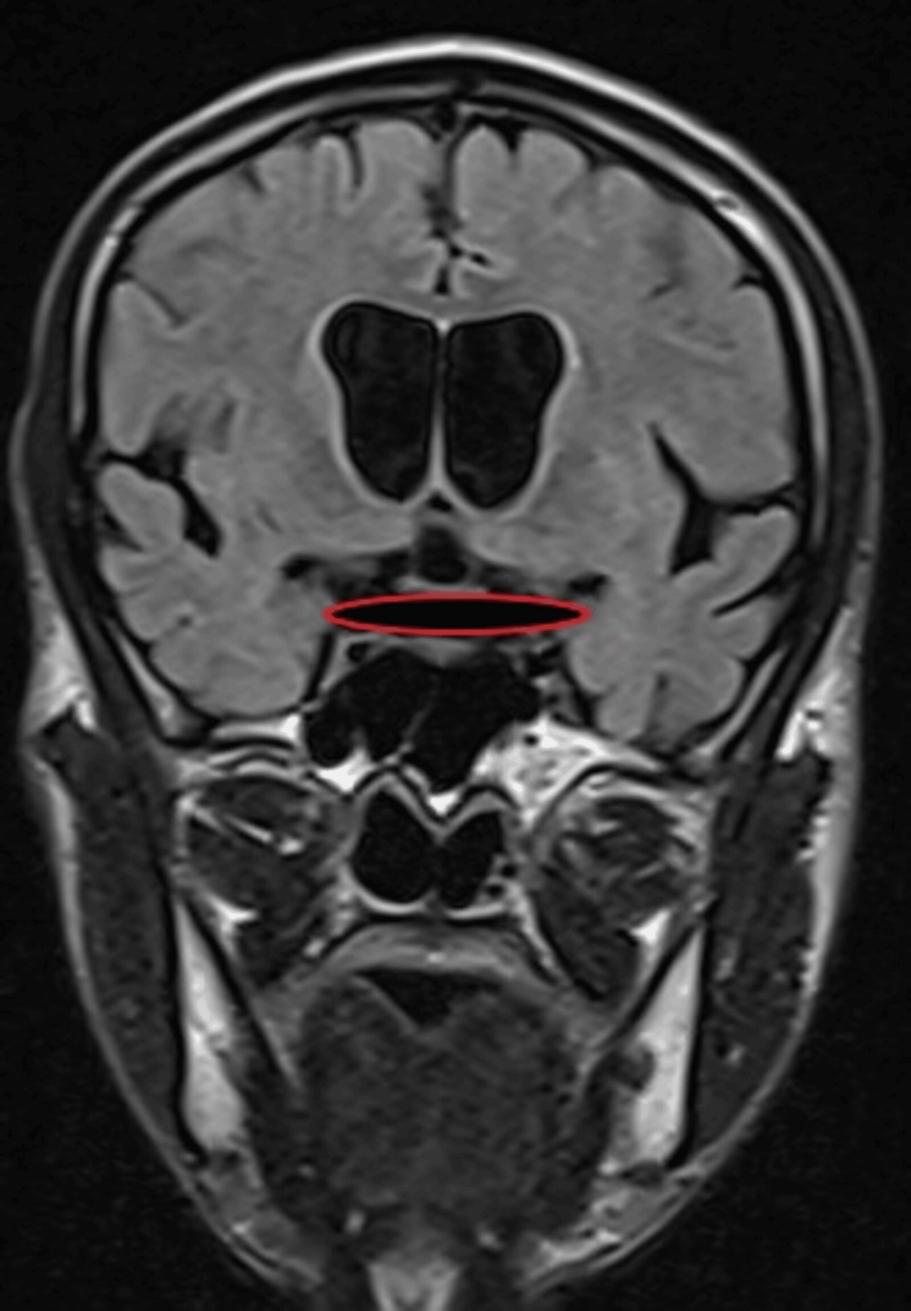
MRI of the brain in T2 FLAIR coronal section showing optic chiasma atrophy (red circle). MRI: Magnetic resonance imaging; FLAIR: Fluid attenuated inversion recovery
